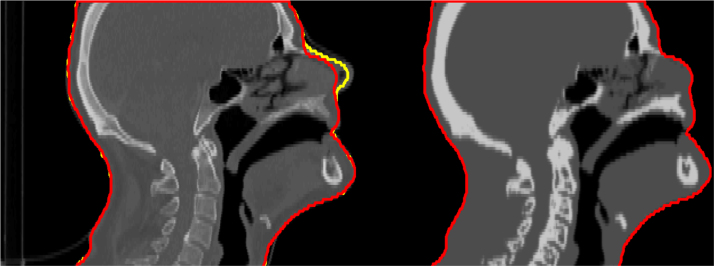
Anonymisation of CT. The left panel shows original CT with original patient body contour in yellow. Both the original CT and body contour could be abused to reconstruct the facial profile. The red contour smooths distinctive properties of the nose, forehead and mouth. To further prevent reverting the anonymisation process, the CT is flattened (right panel).
